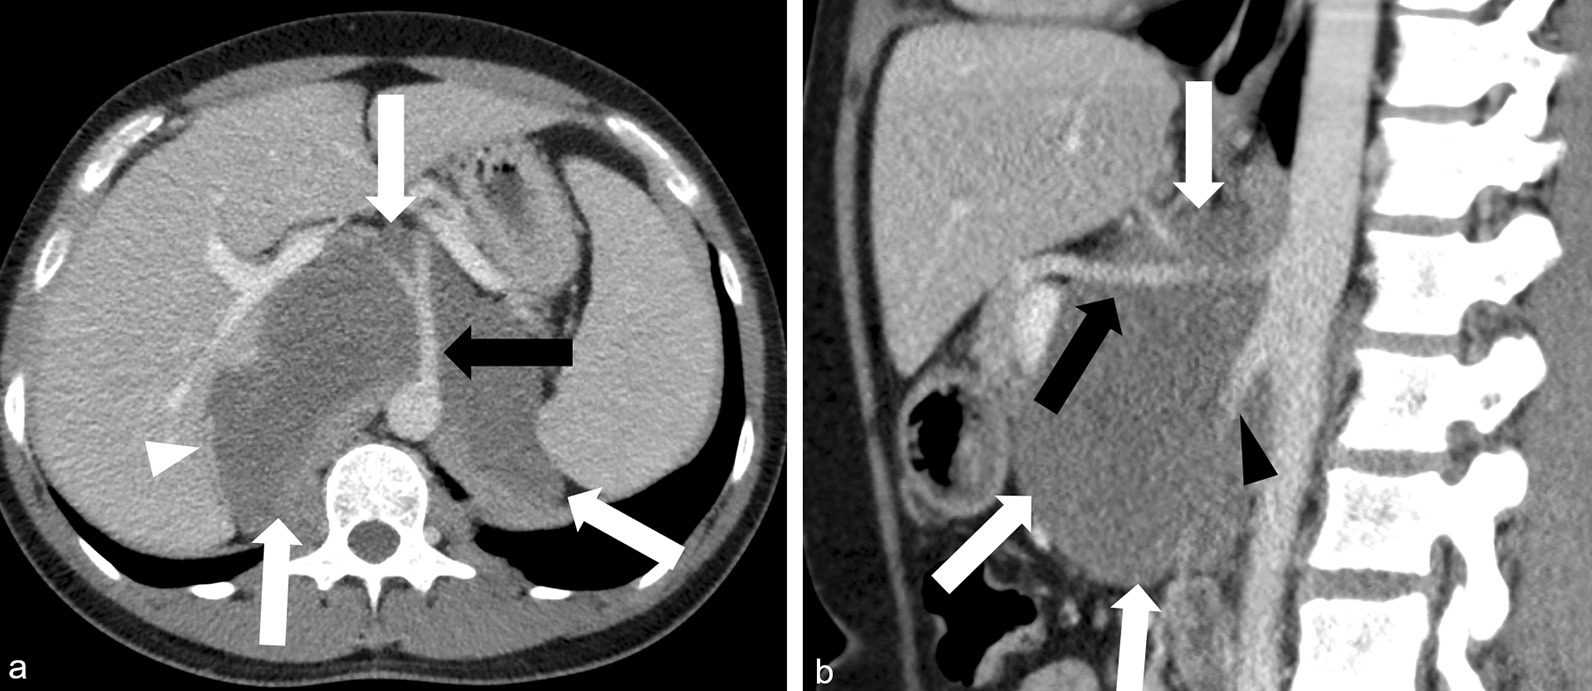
Axial (a) and sagittal (b) contrast-enhanced computed tomography of the abdomen demonstrates a large well-circumscribed hypodense mass in the retroperitoneum (white arrows), with involvement of the right adrenal gland (white arrowhead), encasement of the celiac axis and its branches (black arrows), and encasement of the superior mesenteric artery (black arrowhead)
